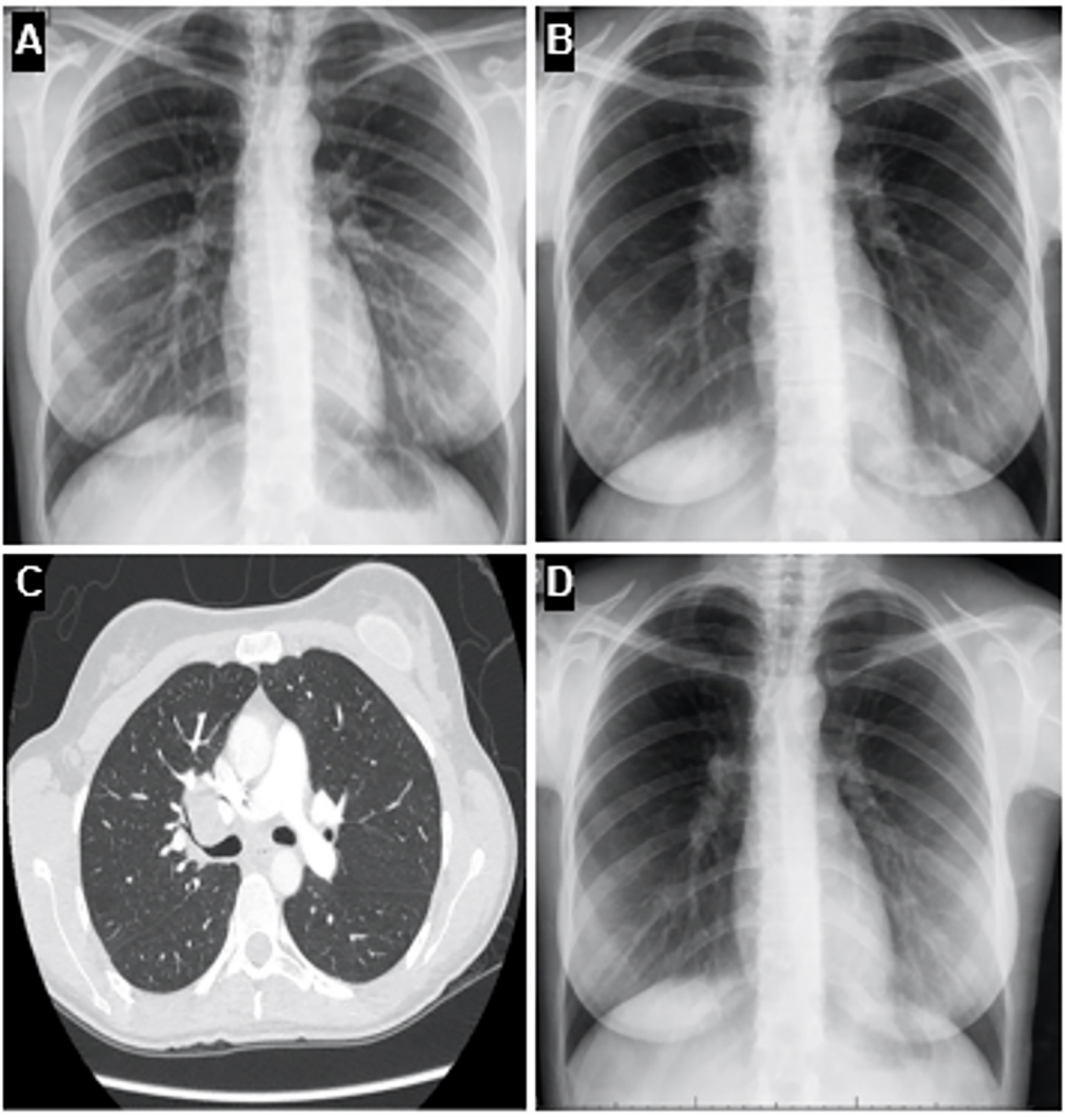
Serial chest imaging: (A) Upon initial diagnosis of Behçet’s disease; (B) During recurrence of oral ulcers, with concurrent fever and weight loss, showing right hilar enlargement; (C) Axial chest CT demonstrates enlarged paratracheal and subcarinal lymph nodes compressing the right main bronchus; (D) Post-completion of anti-tuberculosis therapy.
